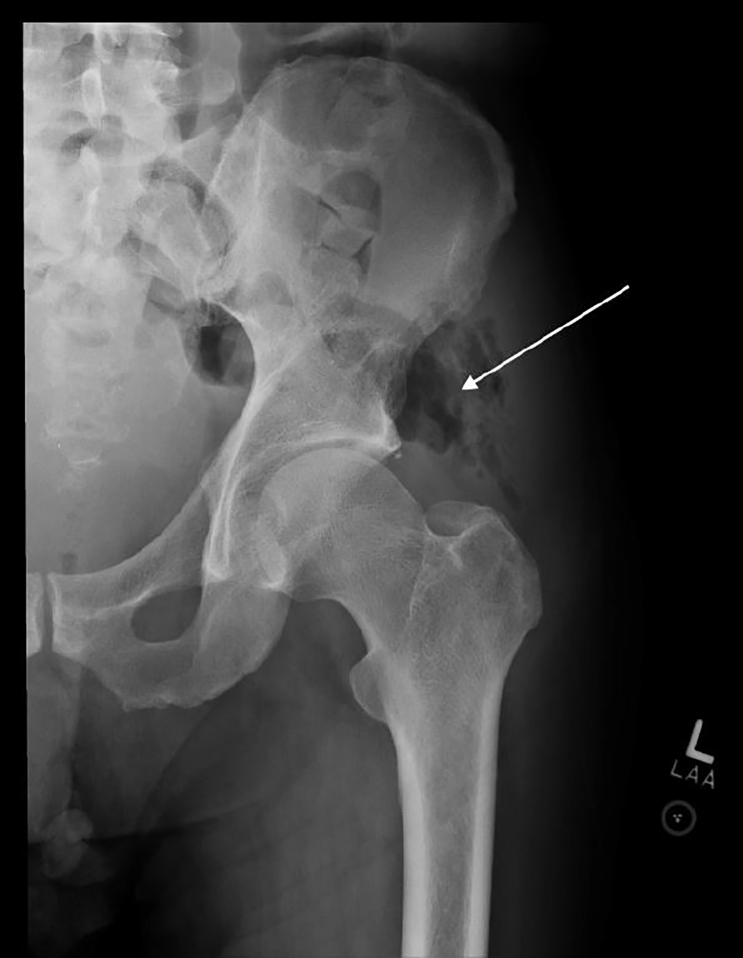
Anterior-posterior left hip radiograph demonstrating free air (arrow) overlying the left acetabulum.
